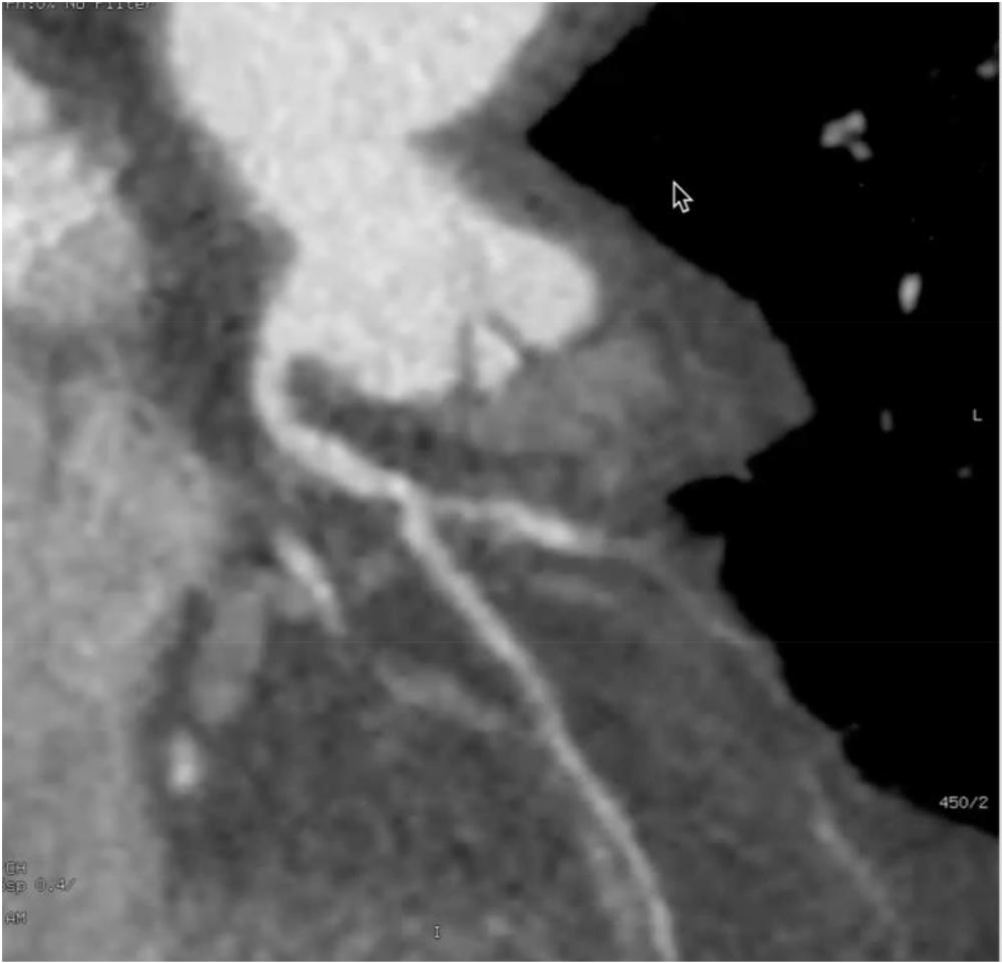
Cardiac computed tomography findings: the right facing cusp gives rise right coronary artery; the proximal segment prior to its bifurcation has moderate to severe coronary artery disease. Coronary artery calcium score is 100.
